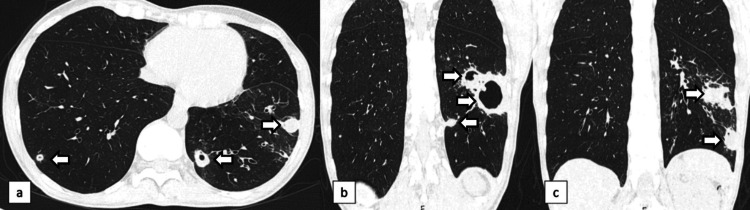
CT scan after four weeks of intravenous antibiotic therapy and Voriconazole CT scan showing cavitated nodules in both lungs, seen in axial (image a) and coronal (images b and c) sections. CT, computed tomography
